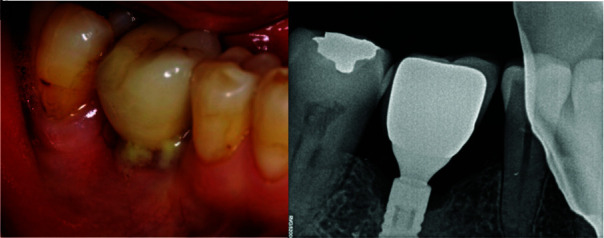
a: Buccal view of #30 with a 7mm probing depth and suppuration on the facial surface, b: Initial radiograph with a crater-like defect and 40% bone loss around #30
